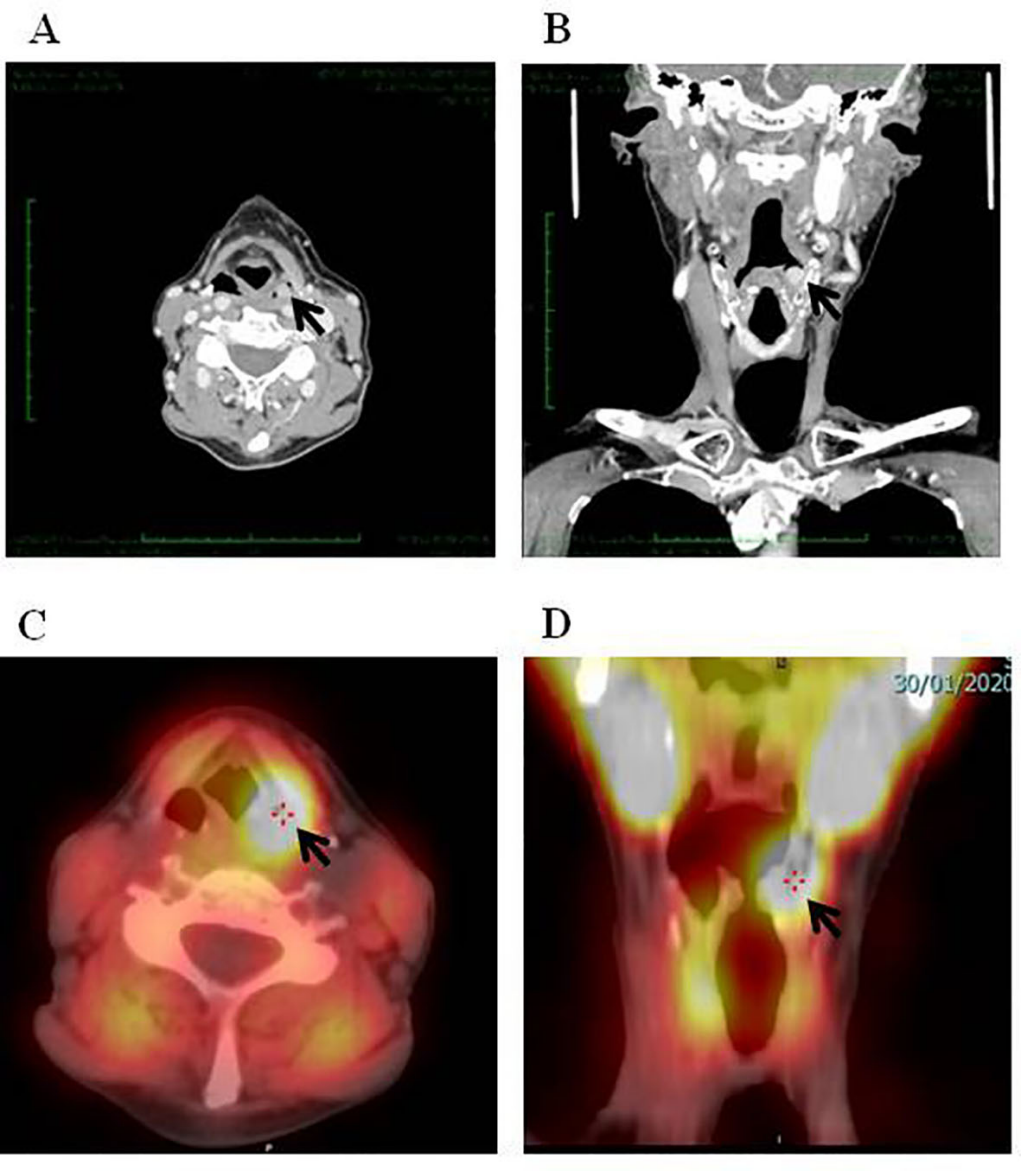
Parathyroid adenoma of the left pyriform identified by neck CT (A, coronal; B, axial) and ^99m^TC-MIBI SPECT/CT (C, coronal; D, axial).
